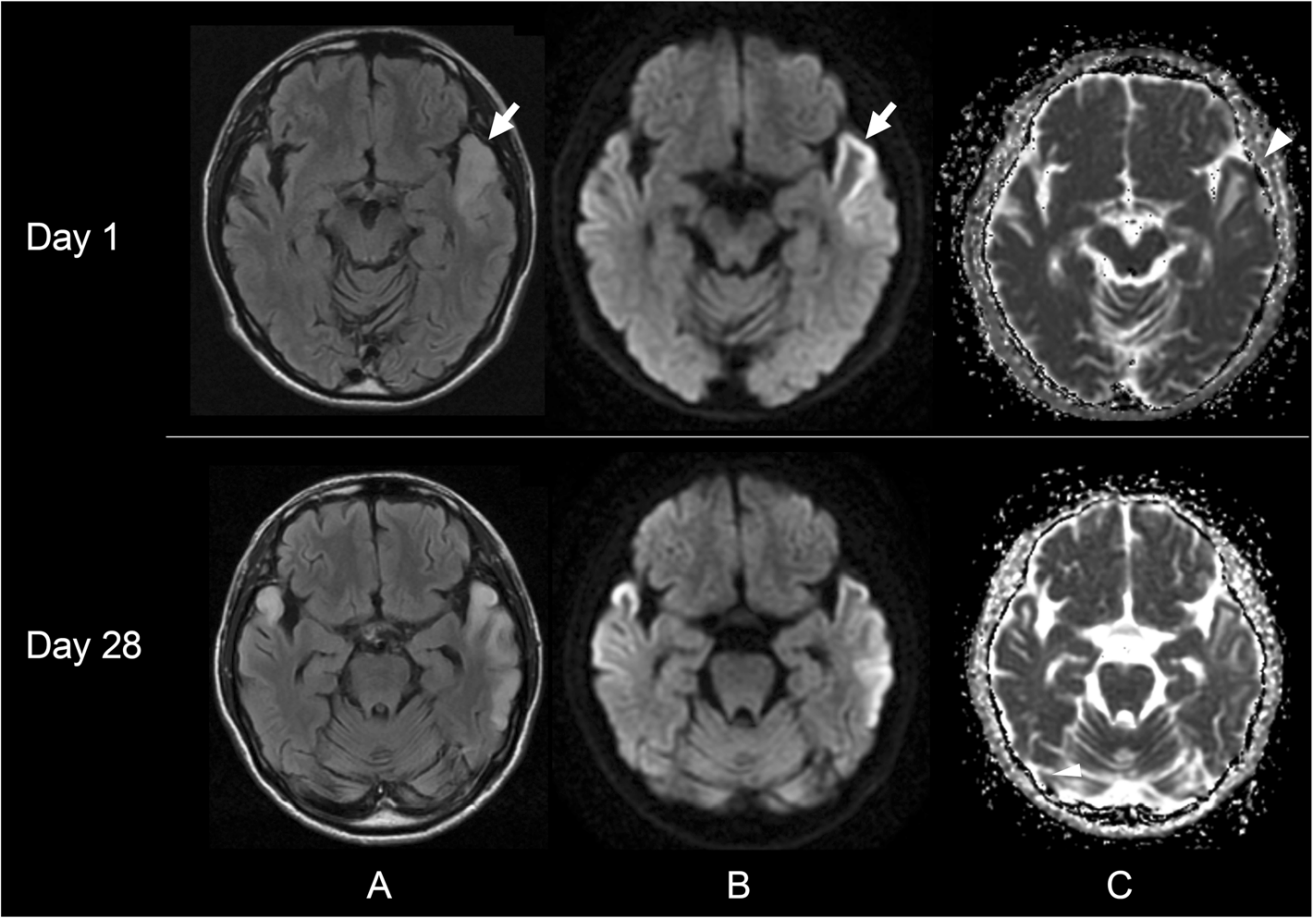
Brain MRIs obtained 14 days after the onset of initial symptom. A brain MRI obtained on admission (day 1) shows increased DWI/FLAIR signal in the left anterolateral temporal lobe, mainly along the cortex with edema (a, b, arrows). The follow-up brain MRI obtained on day 28 shows a newly appearing increased DWI/FLAIR signal in the right anterior temporal lobe, and posterior spread of the initial left temporal lesion along the cerebral cortex (compatible with classic stroke-like lesion). Note mild reduction in ADC values in the left anterior temporal cortex (c, arrowhead), suggesting ongoing regional metabolic derangements. ADC values in the left anterior temporal subcortical white matter is rather increased, suggesting the presence of vasogenic edema. A: DWI; B: FLAIR; C: ADC map
